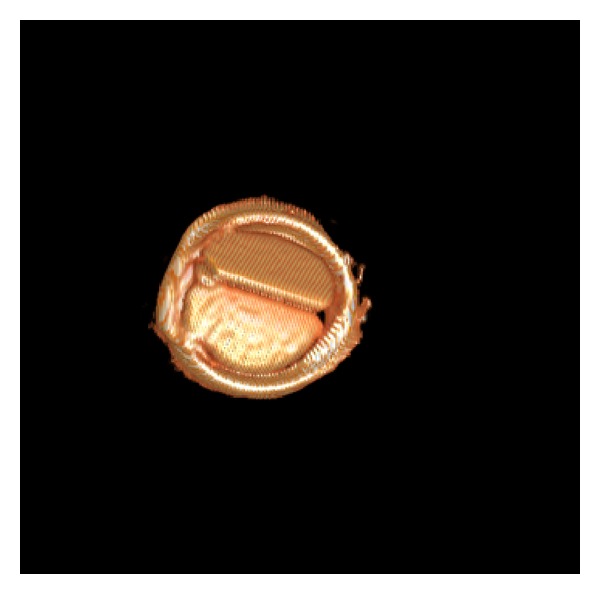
Metallic mechanic prosthetic valve by CT*: 3-D VRT reconstructions permit the evaluation of leaflet dysfunction and valve obstruction. Here: St. Jude mechanic aortic valve, closed during diastole (normal finding). These valves cause artifacts of echo; hence, CT can act as troubleshooter in case of suspected dysfunction or infection.
